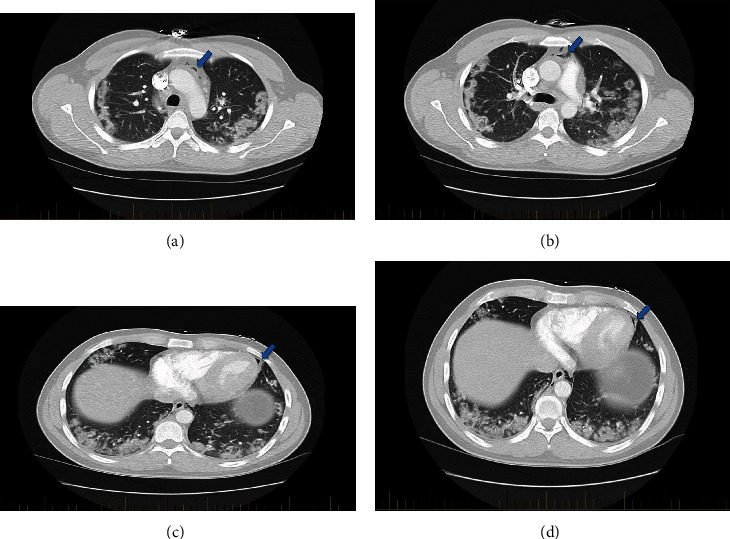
(a) Computed tomographic scans of the chest demonstrating bilateral peripheral ground-glass opacities along with pneumomediastinum (blue arrow). (b) Computed tomographic scans of the chest demonstrating bilateral peripheral ground-glass opacities along with pneumomediastinum (blue arrow). (c) Computed tomographic scans of the chest demonstrating bilateral peripheral ground-glass opacities along with pneumopericardium (blue arrow). (d) Computed tomographic scans of the chest demonstrating bilateral peripheral ground-glass opacities along with pneumopericardium (blue arrow).
